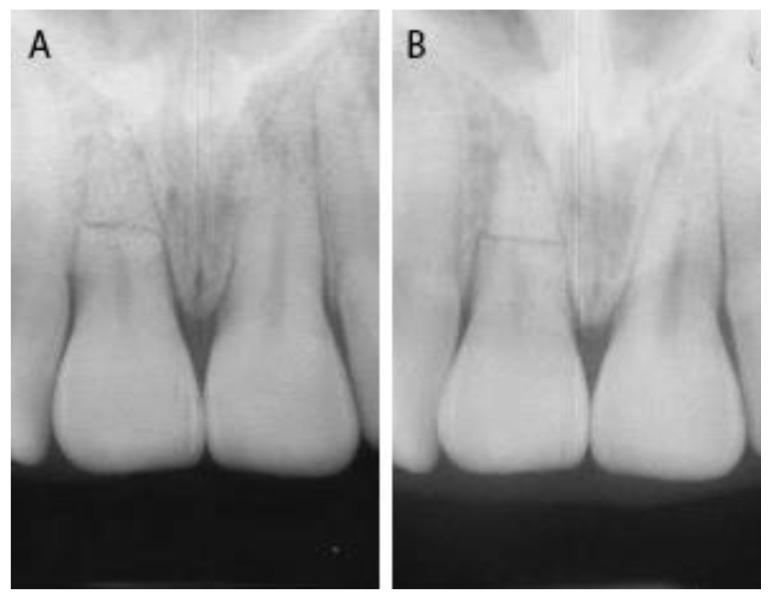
Follow-up, (A) periapical X-ray at three-month recall visit after splint removal and (B) periapical X-ray at twelve-month recall visit after splint removal.
